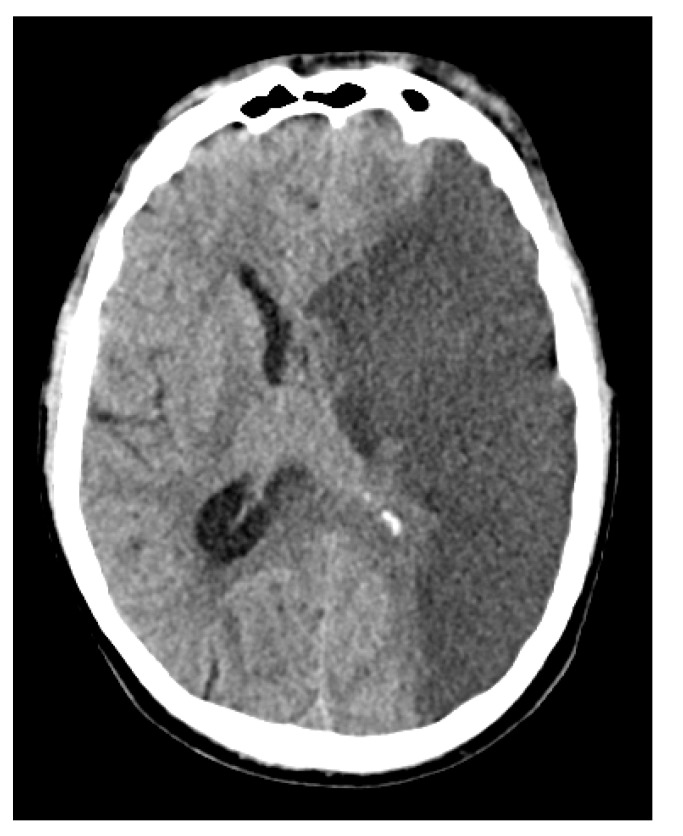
Non-contrast CT scan of the head demonstrating a hypodense area in the left middle cerebral artery territory, indicative of malignant media infarction. The left lateral ventricle is partially compressed, and there is a midline shift of 1–2 cm to the right, suggesting mass effect due to the infarction. No hemorrhagic transformation is seen.
